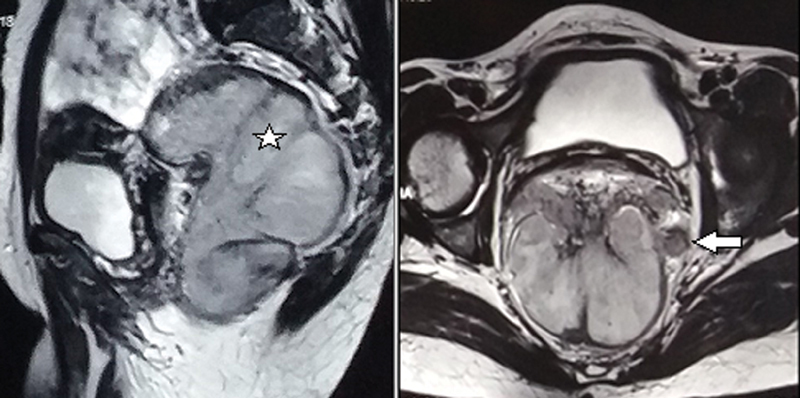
Sagittal and axial sections of MRI pelvis showing a large lobulated mass (★) almost occupying the entire rectal lumen with multiple enlarged perirectal nodes (arrow). MRI, magnetic resonance imaging.
